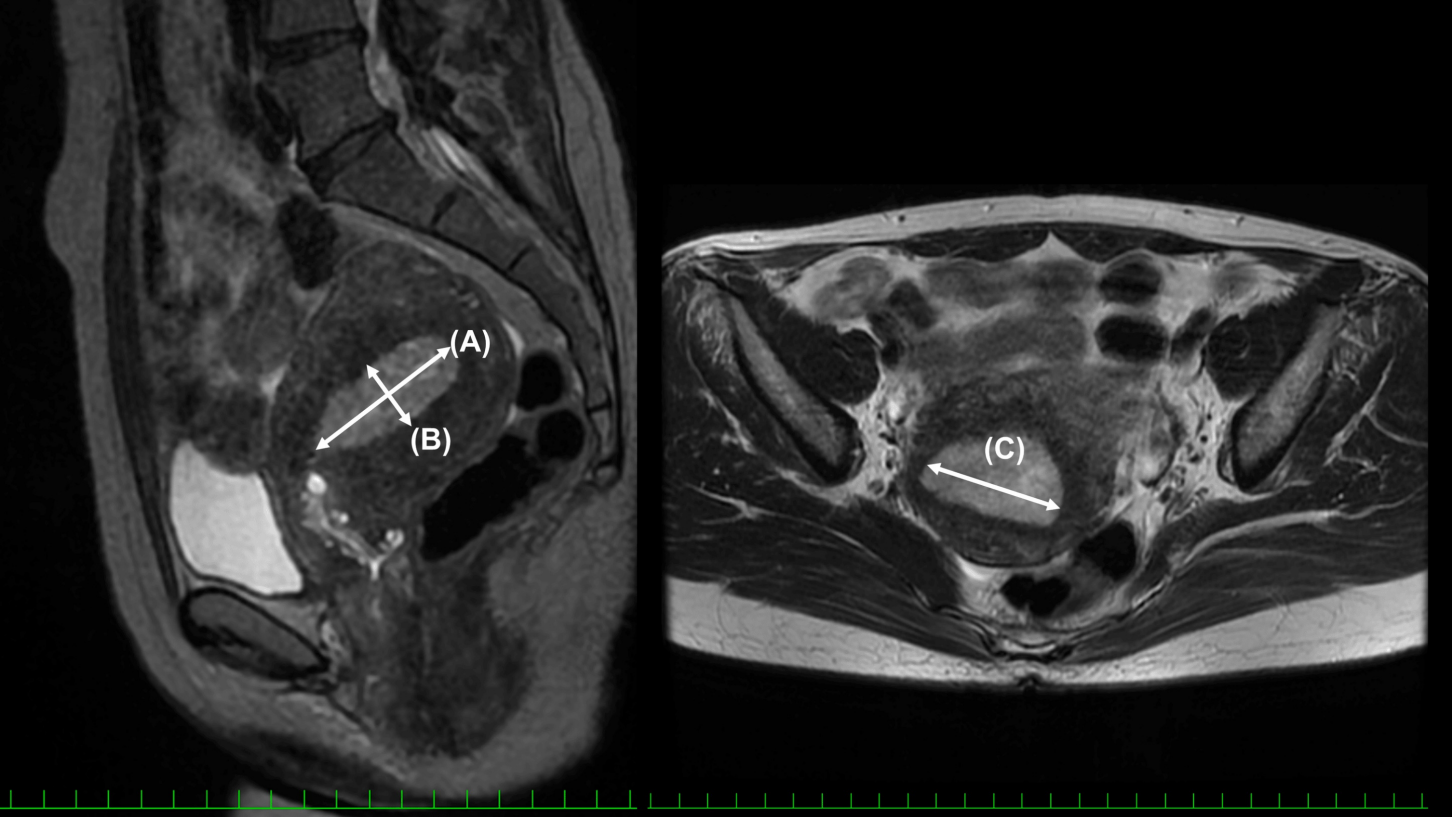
Tumor volume index. The tumor volume index is defined as the product of three measurements on T2-weighted MRI. A: the maximum longitudinal diameter along the uterine axis; B: the maximum anteroposterior diameter on the sagittal plane; and C: the maximum transverse diameter on the horizontal plane. The representative images (original to this article) demonstrate the method used to calculate the tumor volume index as described in [5].
